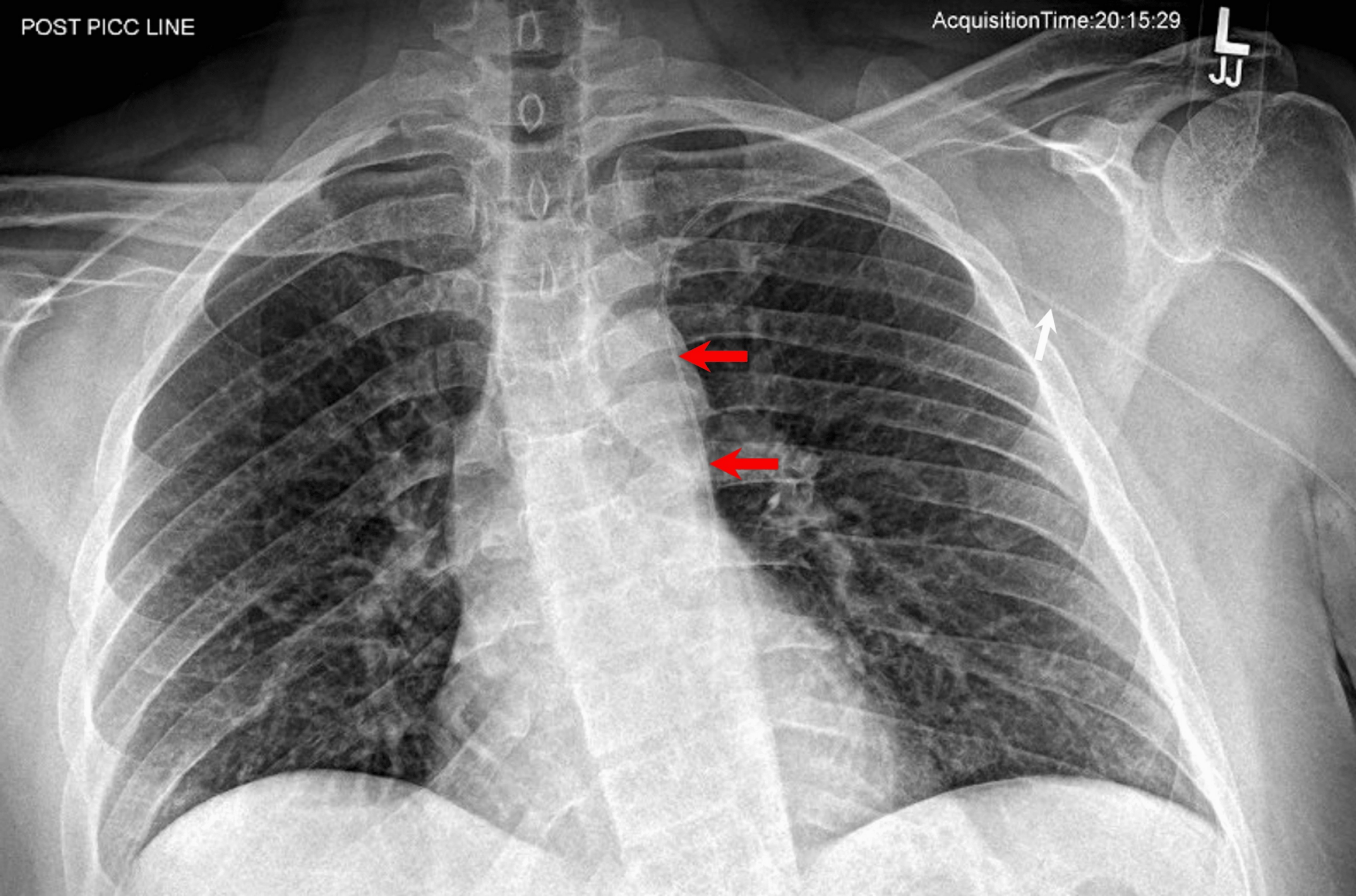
Anteroposterior X-ray of the chest Note that the peripherally inserted central catheter (red arrows) in the left upper extremity does not cross to the right of midline to the expected location of the superior vena cava. It courses centrally to the expected location of the left subclavian vein, but then turns inferiorly into the left side of the mediastinum.
